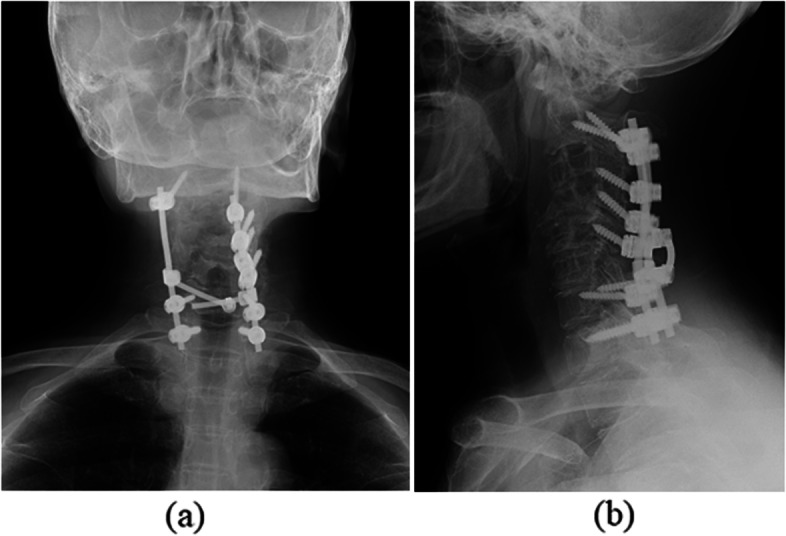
Postoperative radiographs. We performed posterior decompression and fixation from C2 to T1 and partial tumor resection to improve the patient’s cervical myelopathy symptoms
